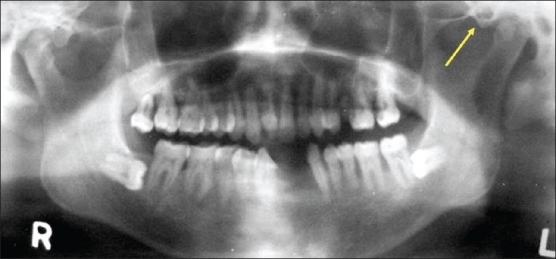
Panoramic radiograph shows a unilateral (L) multilocular ZACD (arrow)
